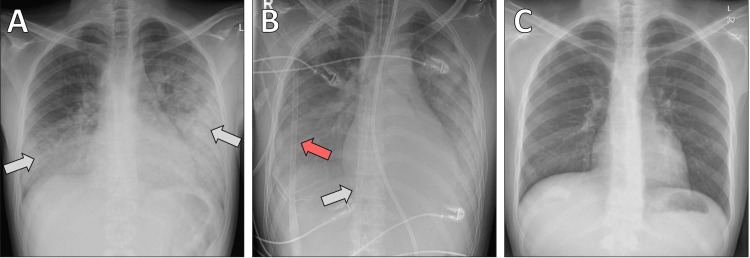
Chest X-ray findings A: on admission, chest radiograph demonstrating advanced bilateral pulmonary edema (white arrows); B: after central implantation of the extracorporeal life support (ECLS) system, arterial cannula in the right axillary artery (red arrow) and venous cannula inserted via the right common femoral vein, extending into the superior vena cava (white arrow); C: on discharge, chest radiograph showing complete resolution of pulmonary pathology with normal findings.
